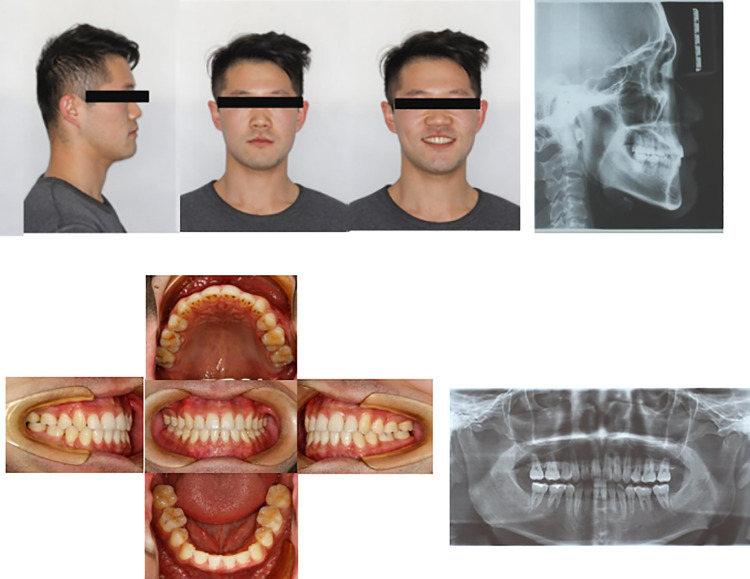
Posttreatment facial, intraoral photographs, and radiographs of the case with premolar tooth extraction. These showed that bilateral molar relationships were neutral, with normal overbite.
